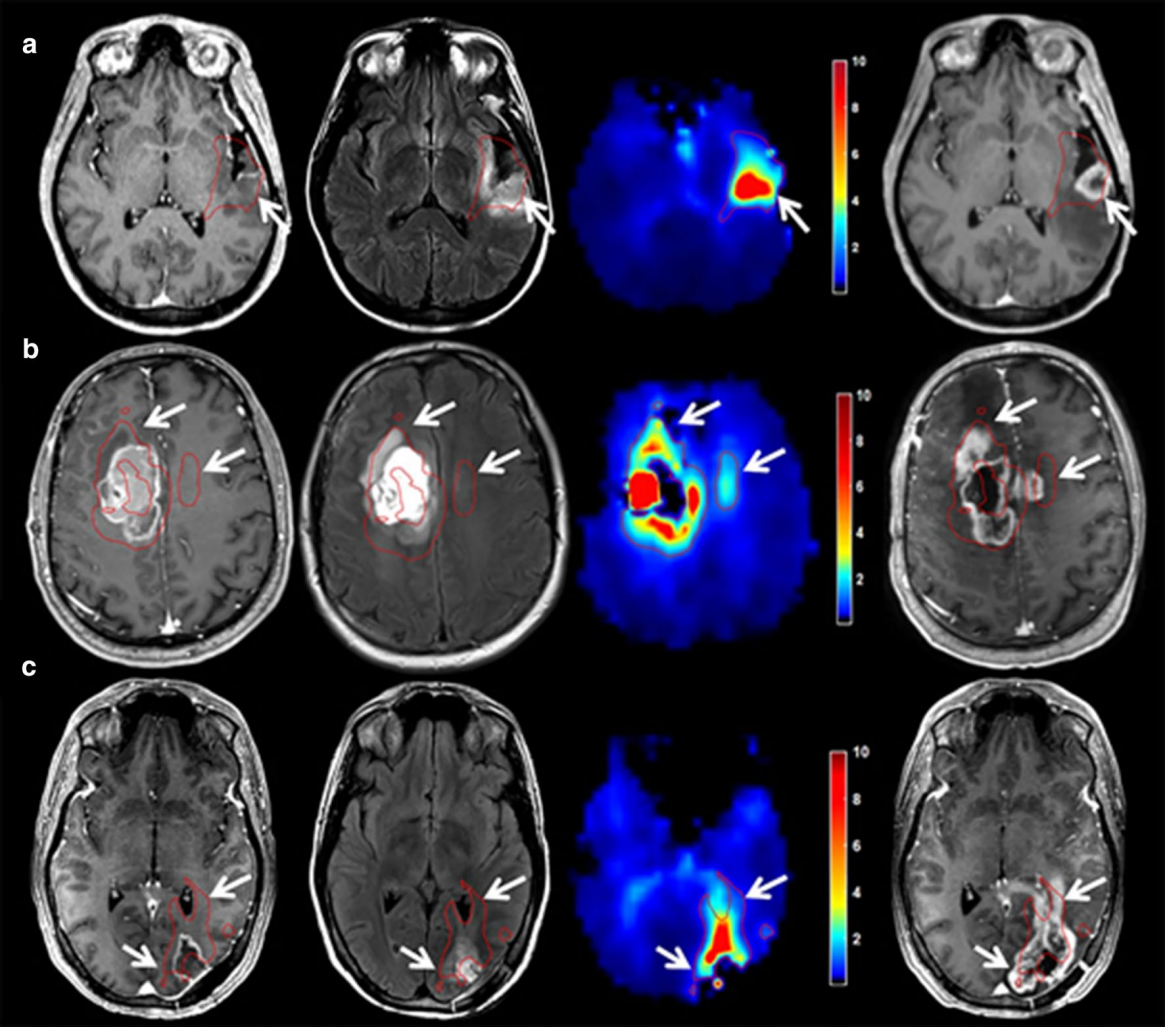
Pre-radiotherapy Cho/NAA abnormalities were observed and delineated (red contour). Pre-RT imaging shows that increased Cho/NAA ratios on the Cho/NAA map (third column) extended beyond the GTV on T1-weighted contrast enhancement (first column) and T2-weighted/FLAIR (second column). First recurrence on contrast-enhanced T1-weighted imaging after radiotherapy (fourth column) showed contrast-enhancing tumour development within the pre-radiotherapy increased Cho/NAA volume in 3 patients (a–c) (reprinted with permission from Cordova et al*.* [93])
